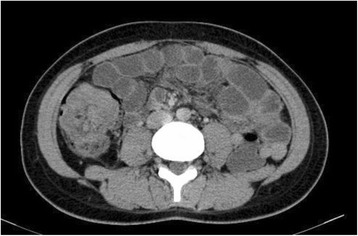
Axial CT showing intussusception at the ascending colon with wall thickening
